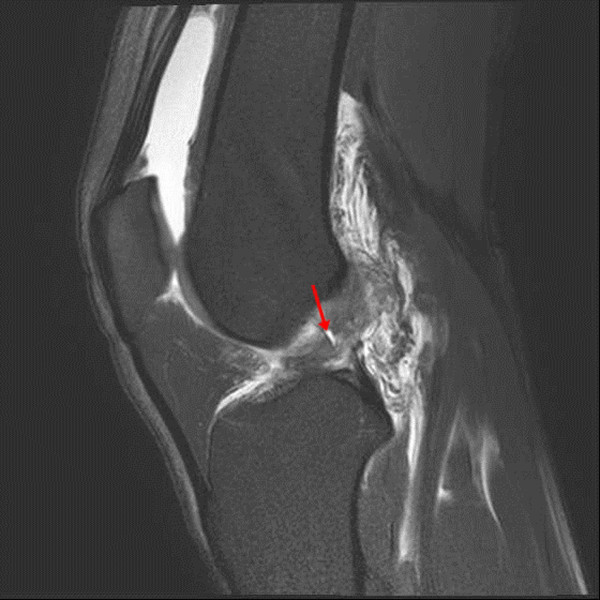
In this T2-weighted fat-suppressed sagittal image, abnormal signal intensity is observed as increased signal intensity within the anterior cruciate ligament (arrow).
